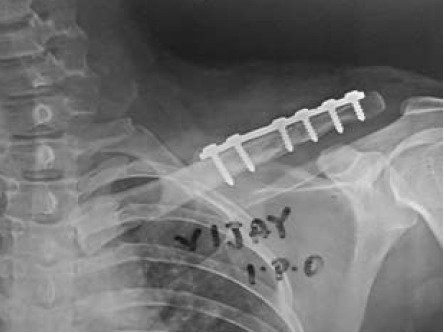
Fig. 5: Sixth month post- operative radiograph. F5:
